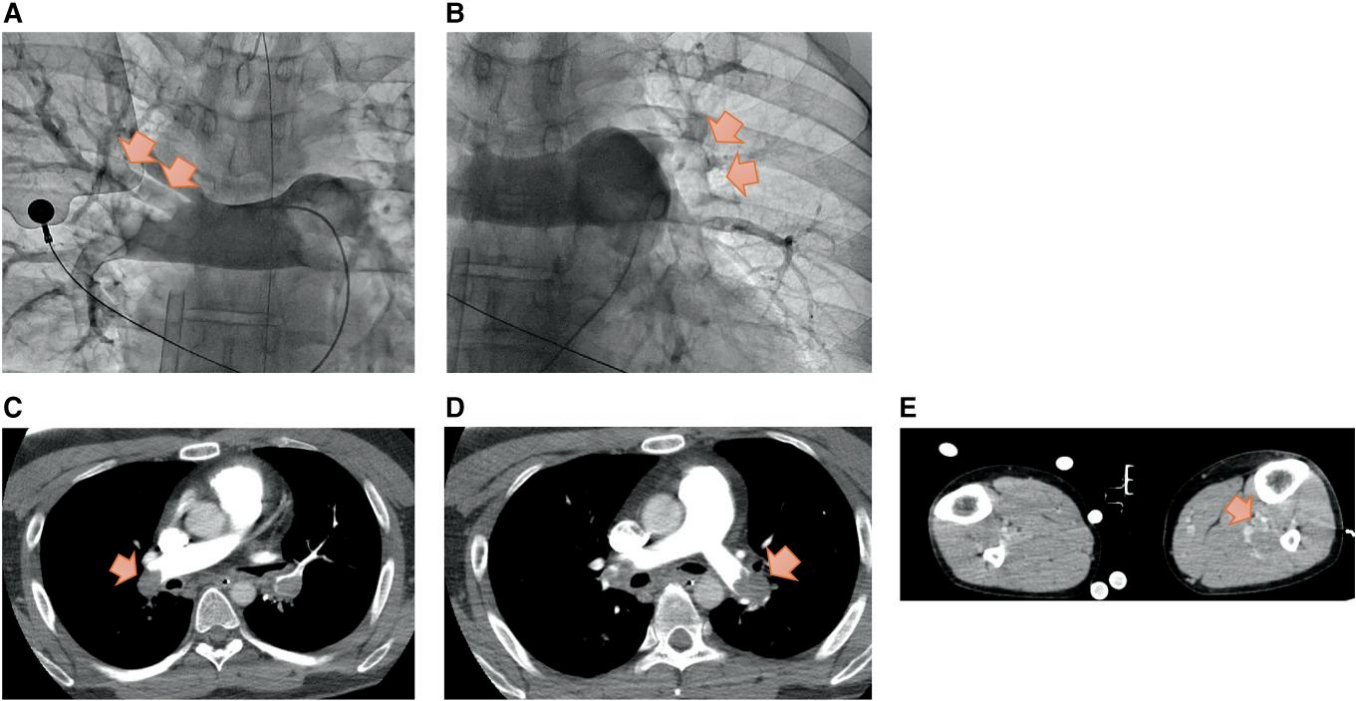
Imaging findings on admission. (*A*, *B*) Pulmonary angiography images and (*C–E*) computed tomography images showed thrombi in the proximal portions of both pulmonary arteries and in the posterior tibial and fibular veins (arrows).
